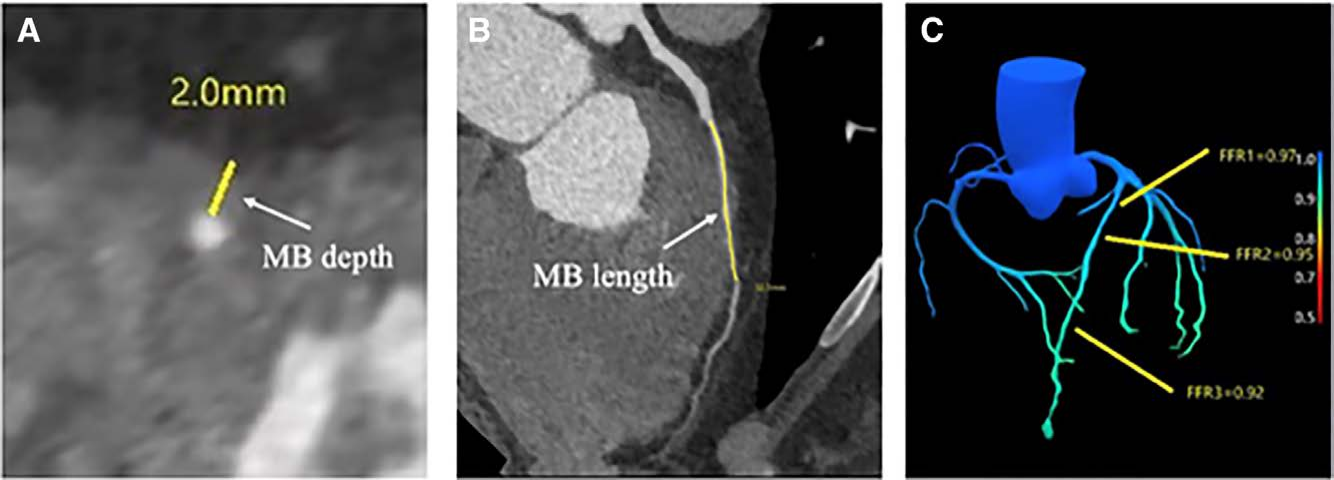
CCTA and FFR_CT_ features of the left anterior descending coronary artery with myocardial bridging. Deep MB in the mid-segment of the LAD in a 51-year-old man. (A) The MB depth is 2.0 mm. (B) The MB length is 32.3 mm. (C) The FFR_CT_ values distal to the MB (FFR3) is 0.92. CCTA = coronary computed tomography angiography, FFR_CT_ = CT-derived fractional flow reserve, LAD = left anterior descending coronary artery, MB = myocardial bridging.
